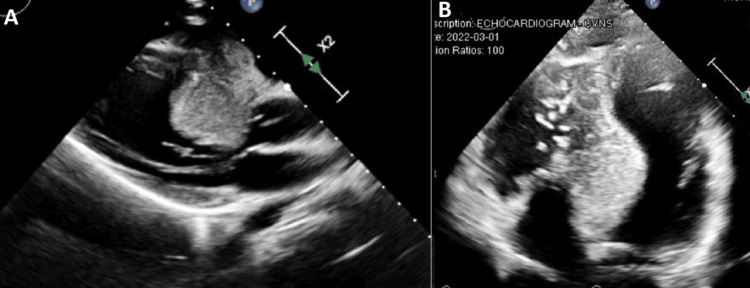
TTE (A) Parasternal long axis view and (B) apical four-chamber view. A large mass in the interventricular septum measuring 5 x 5 x 3 cm in diameter. There appears to be partial obliteration of the interventricular septum. TTE, transthoracic echocardiogram
